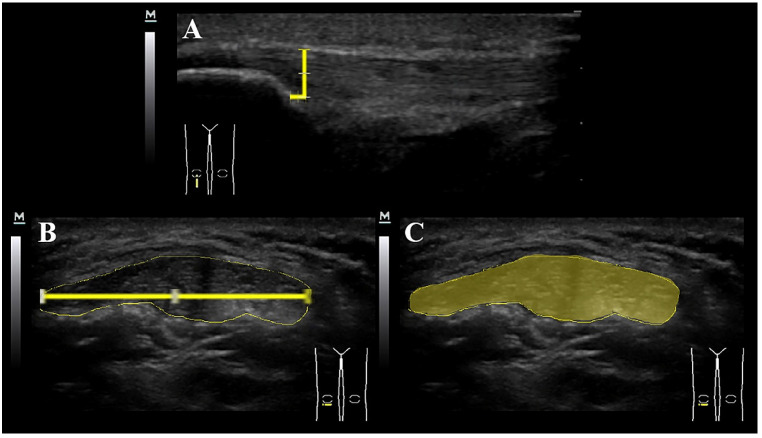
Ultrasound assessment of patellar tendon thickness (A), width (B) and cross-sectional area (C).
